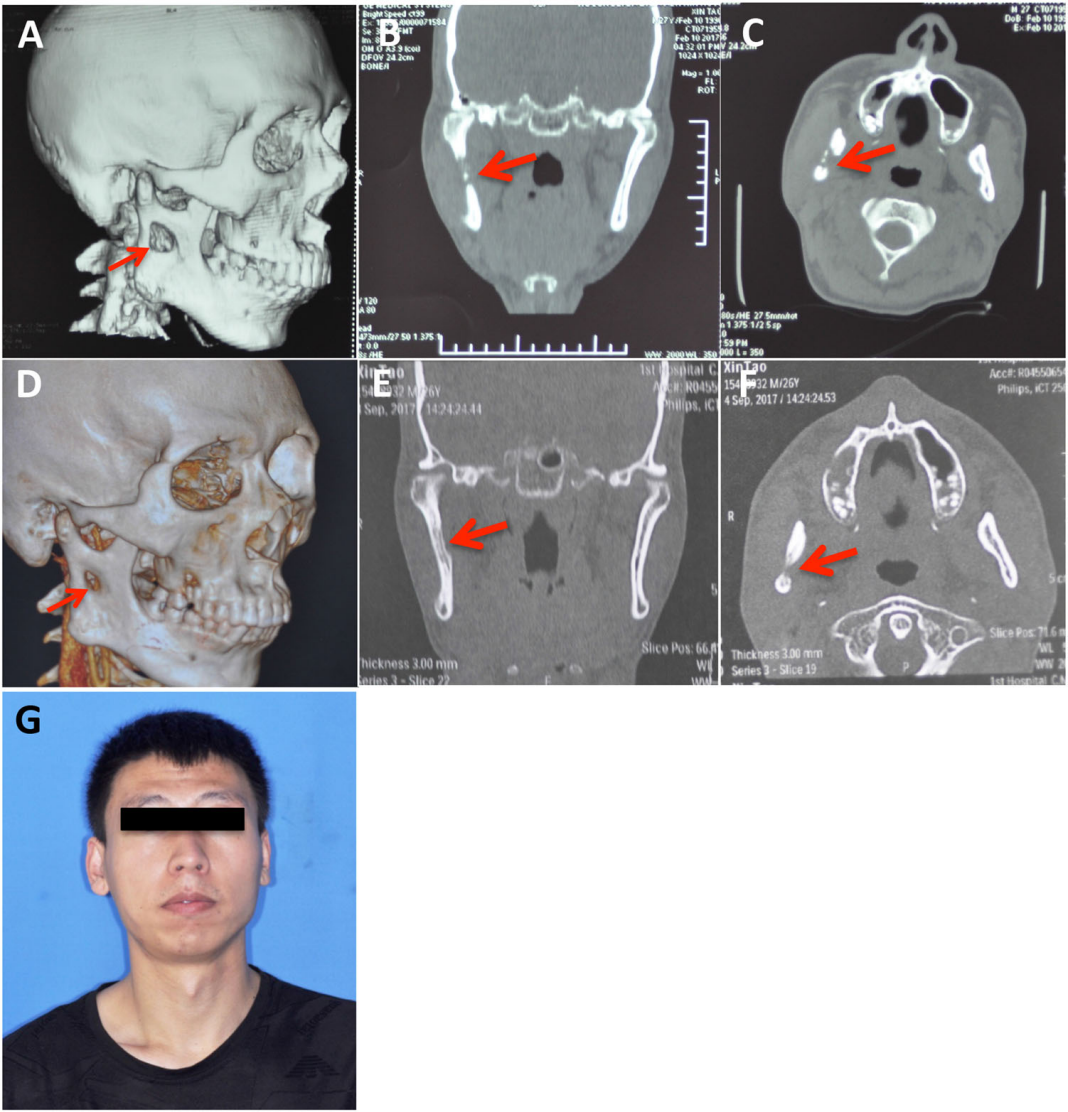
Follow-up material of the HNTB patient—the case report. a–c Computed tomography results after two months of treatment. d–f Computed tomography results after ten months of treatment. g Frontal photograph after ten months of treatment. Red arrow: mandibular involvement
